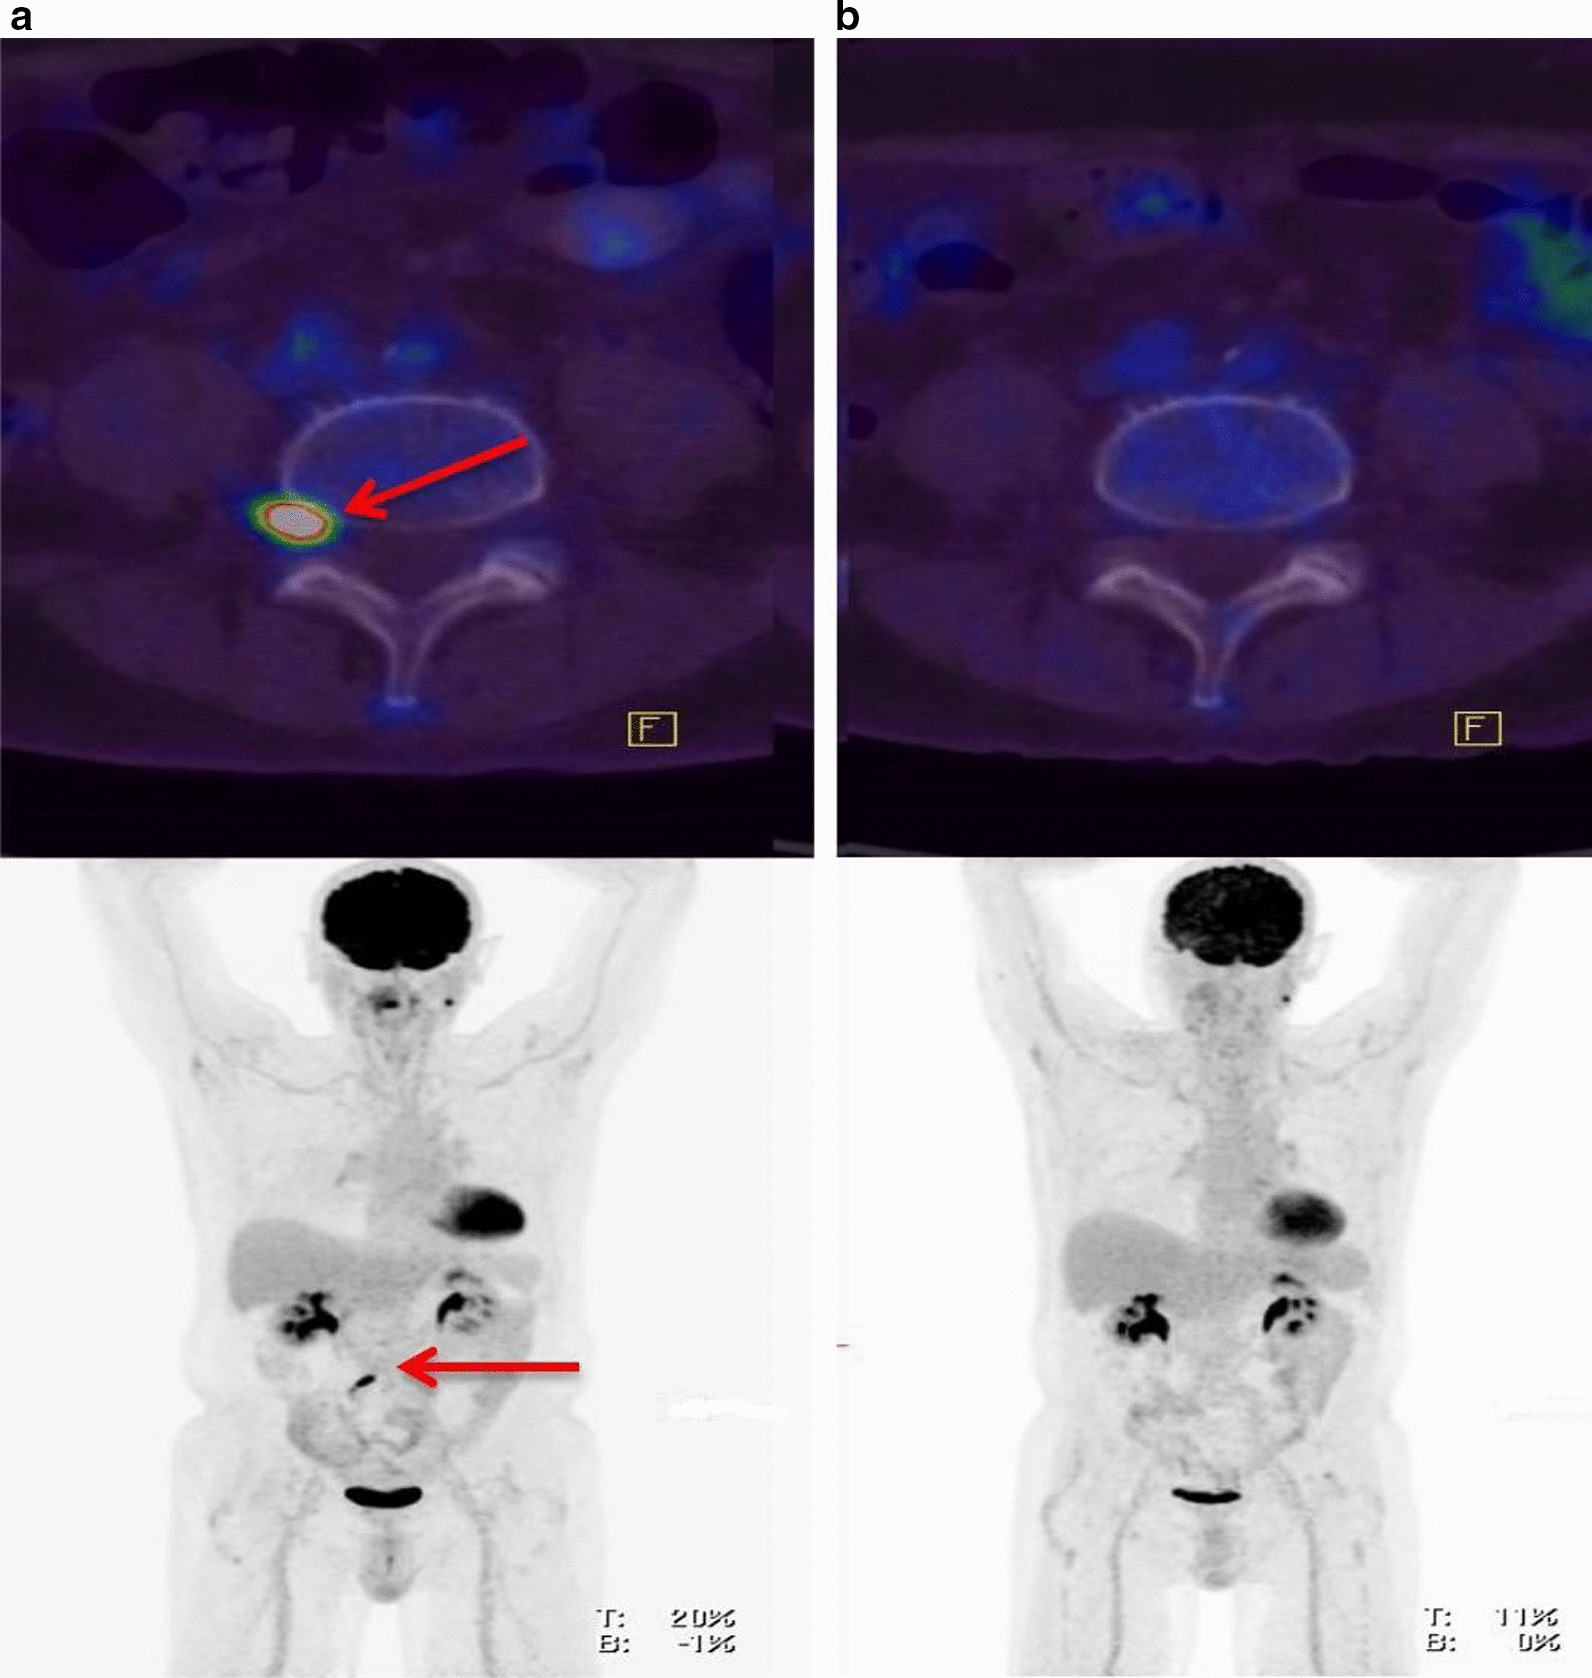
a Intense uptake projected to the right L4 spinal nerve root might represent DLBCL relapse (the red arrow is pointing to L4 spinal nerve lesion). b Post-treatment: compared to the previous PET/CT complete remission of DLBCL is evident. Left-sided benign tumor of the parotid gland is noted with no interval change
